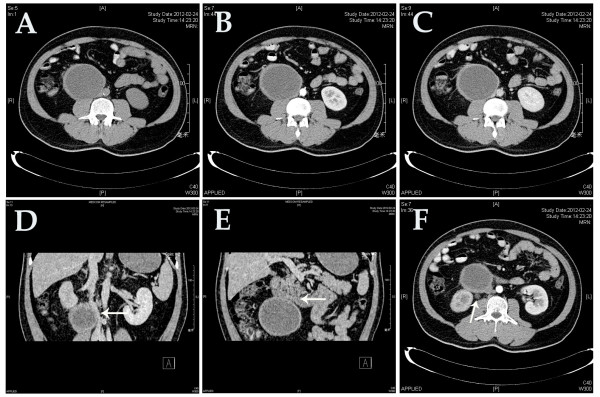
Computed tomography scans. (A–C) Plain scan, artery phase, and portal phase, respectively, showed a cyst-like and well-encapsulated tumor, with mild enhancement of the wall on portal phase. (D–F) Coronal and transverse sections showed that the tumor was compressing the inferior vena cava, duodenum, and right upper ureter respectively (white arrows).
